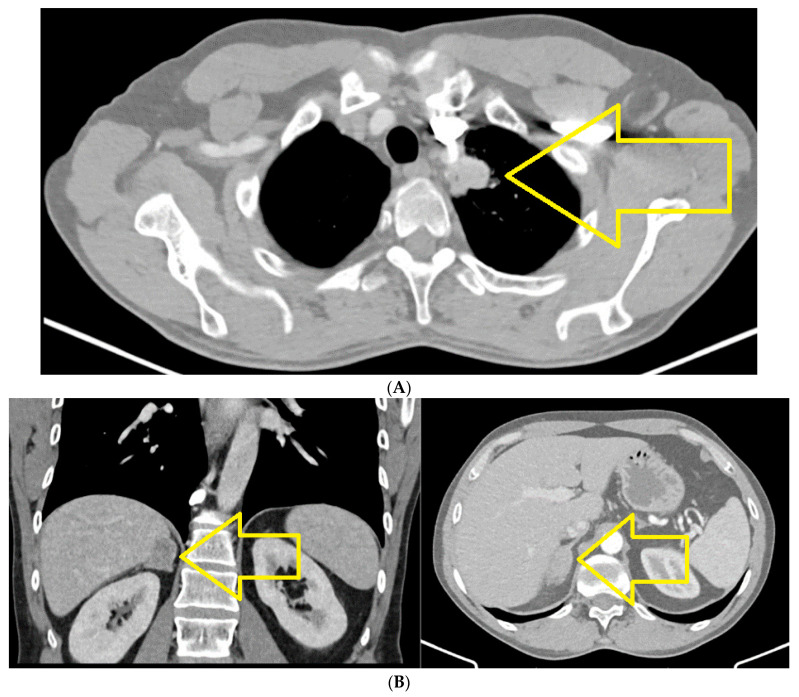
(A) Chest CT August 2021: left upper lobe lung tumor. (B) CT abdomen August 2021: right adrenal node newly appeared (secondary).
